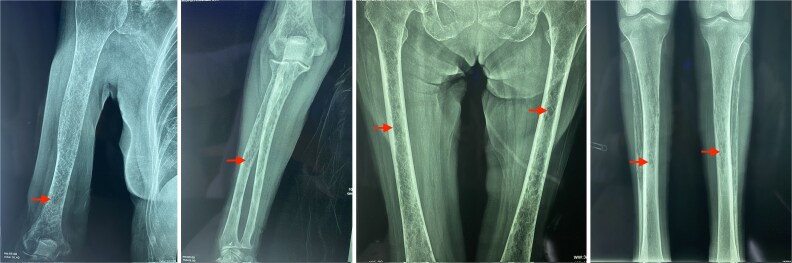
Radiographs of long bones in a 64-year-old woman with oligosecretory multiple myeloma. (A) Right humerus, (B) right ulna and radius, (C) left and right femur, (D) left and right tibia, showing multiple osteolytic lesions (red arrows).
